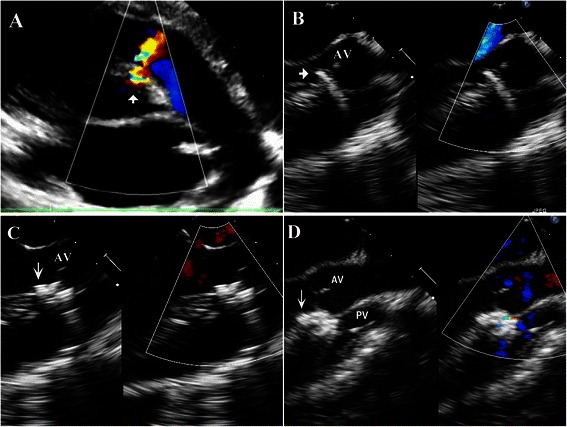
Different steps of perventricular device closure of a small doubly committed ventricular septal defect (DCVSD). a A small DCVSD (*arrowhead*) located at the 1 to 2 o’clock position in the parasternal short-axis view on transthoracic echocardiography. b The delivery sheath (*arrowhead*), which was perpendicular to the septum, was loaded with the device and passed through the defect. c The left disk was opened with its platinum marker (*arrow*) pointing towards the apex. While maintaining gentle tension on the cable without rotation, the delivery sheath was withdrawn to deploy the right disk of the device into the right ventricle. d The eccentric occluder was placed in a proper position without affecting the aortic and pulmonary valves. (AV = aortic valve, PV = pulmonary valve)
